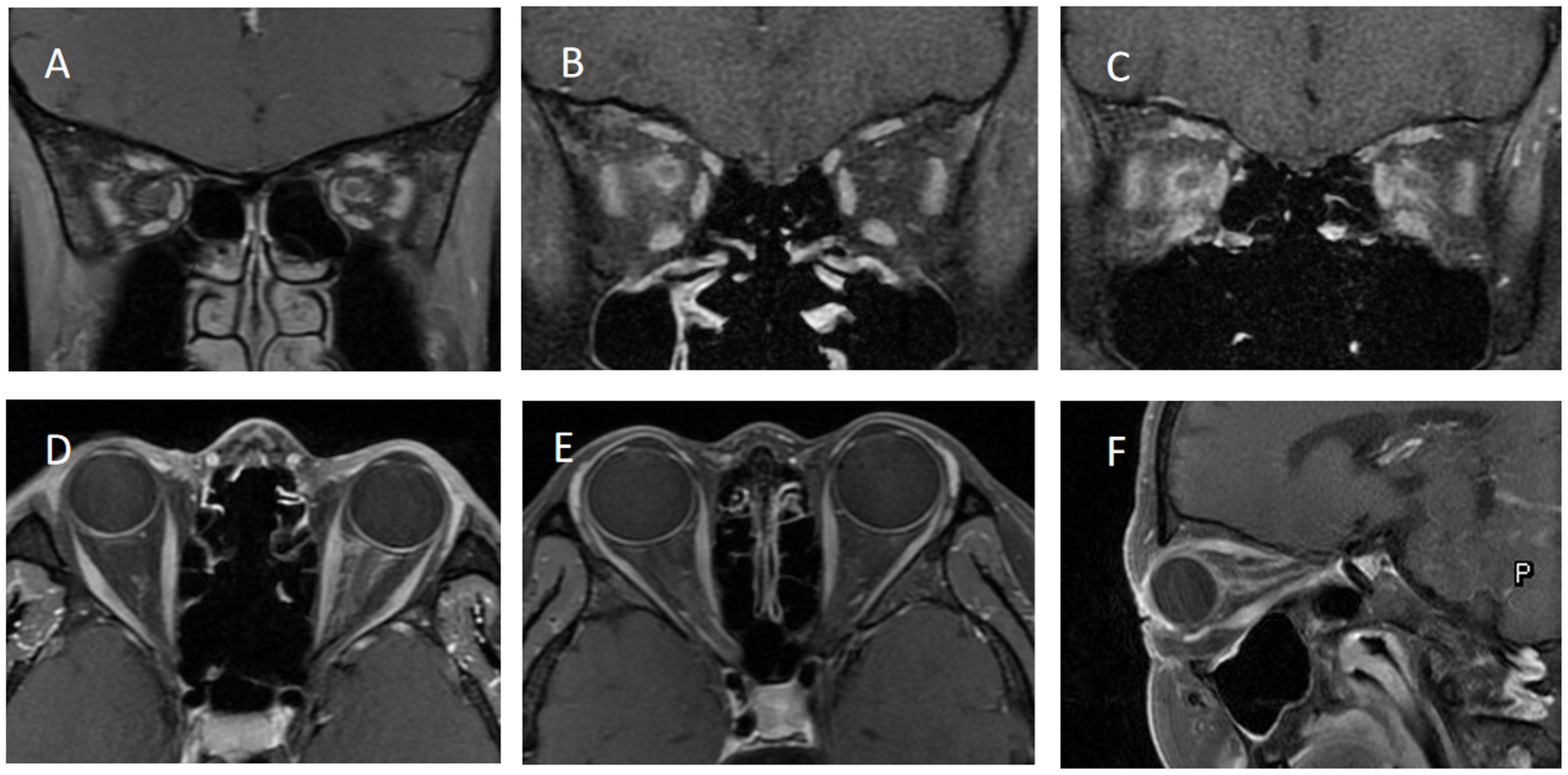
shows the orbital MRI of different optic perineuritis (OPN) patients. (A) The coronal T1 contrast image showed enhancement of the left optic nerve sheath (ONS). (B) Coronal T1 contrast image showed enhancement of the right ONS (C) Coronal T1 contrast image showed enhancement of both ONS. (D) Axial T1 contrast image showed enhancement of left ONS. (E) Axial T1 contrast image showed enhancement of right ONS. (F) The sagittal T1 contrast image showed left ONS.
